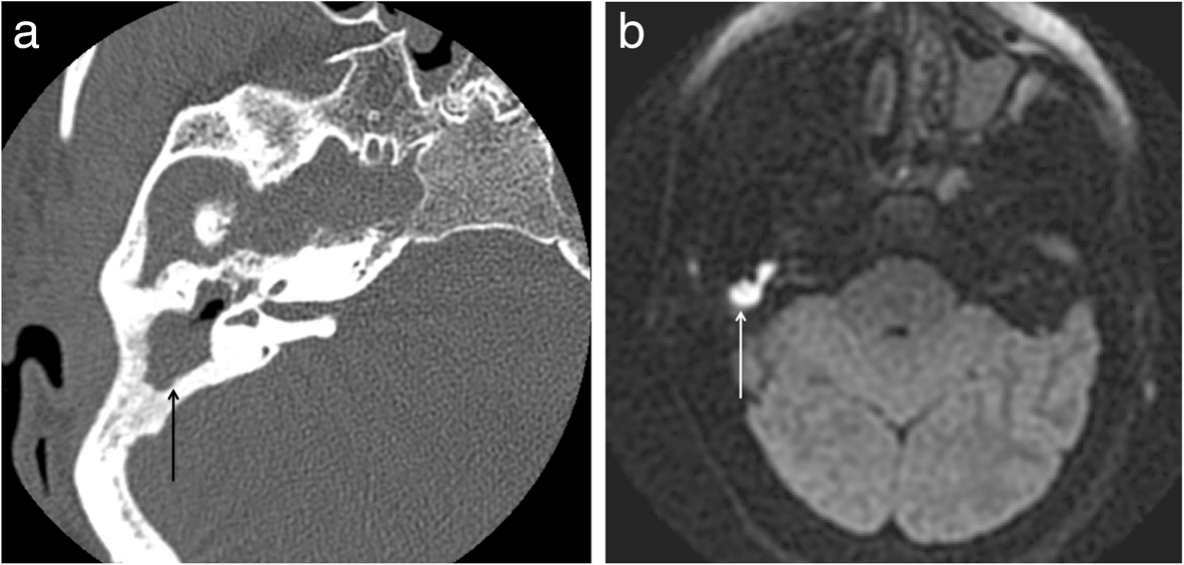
Patient with recurrent cholesteatoma following a canal-wall up tympanomastoidectomy. A) Axial unenhanced small field of view CT image demonstrates low density soft tissue (black arrow) filling the attic, aditus ad antrum and epitympanum. B) Axial 3 mm thick PROPELLER non-EPI DWMRI shows avid restricted diffusion (white arrow) within the recurrent cholesteatoma in the attic and epitympanum
